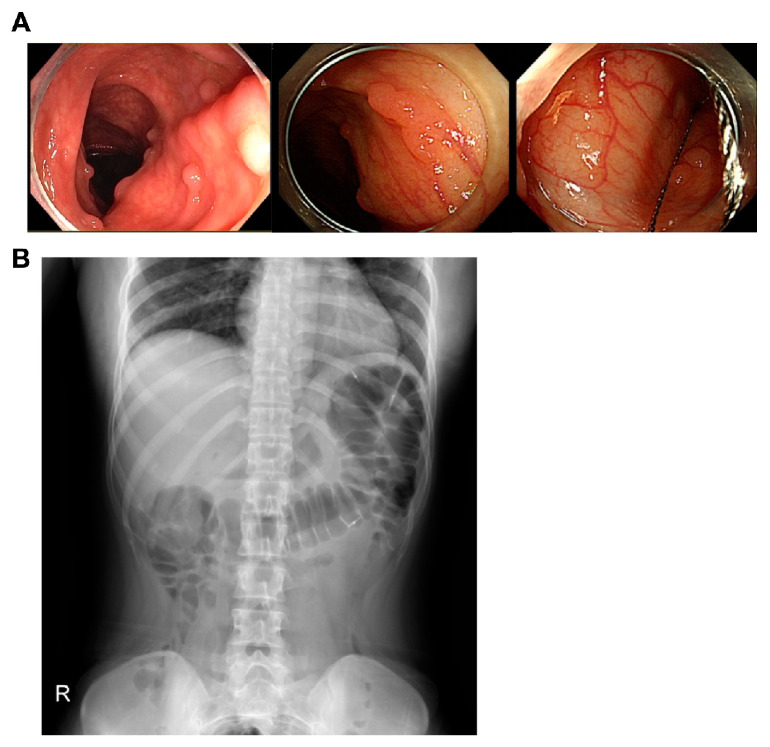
Colonofiberscopy and plain abdominal X-ray of the patient. (A) Colonofiberscopy results showing multiple sessile diminutive colon polyps and the cold-snare polypectomy procedure. (B) Plain abdominal X-ray after the colonoscopy, showing hemoclips marking the anatomical location of the colon polyps.
